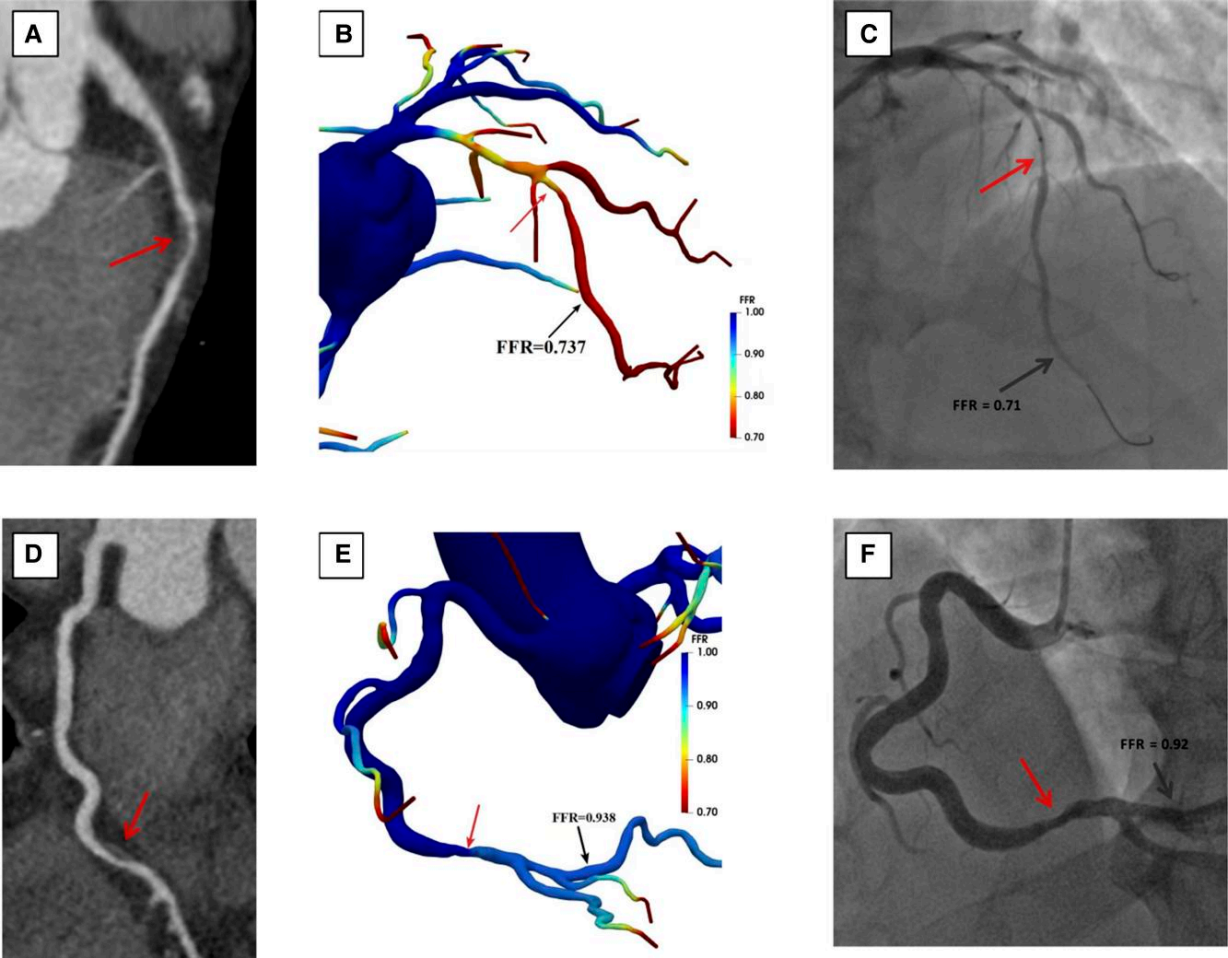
Case example. Two patients both with anatomically obstructive stenosis (arrow) on coronary computed tomography angiography (CCTA) (*A* and *D*). In the first patient (*A–C*), computation of fractional flow reserve from coronary computed tomography angiography (CT-FFR) demonstrates lesion-specific ischaemia of the middle left anterior descending (LAD) stenosis, with a value of 0.74 (*B*). Invasive coronary angiography confirms the functionally obstructive stenosis with an invasive FFR of 0.71 (*C*). In the second patient (*D–F*), CT-FFR predicts no ischaemia of the stenosis in the distal right coronary artery (RCA) with a CT-FFR value of 0.94 (*E*). Invasive FFR of 0.92 similarly demonstrates no ischaemia of RCA.
